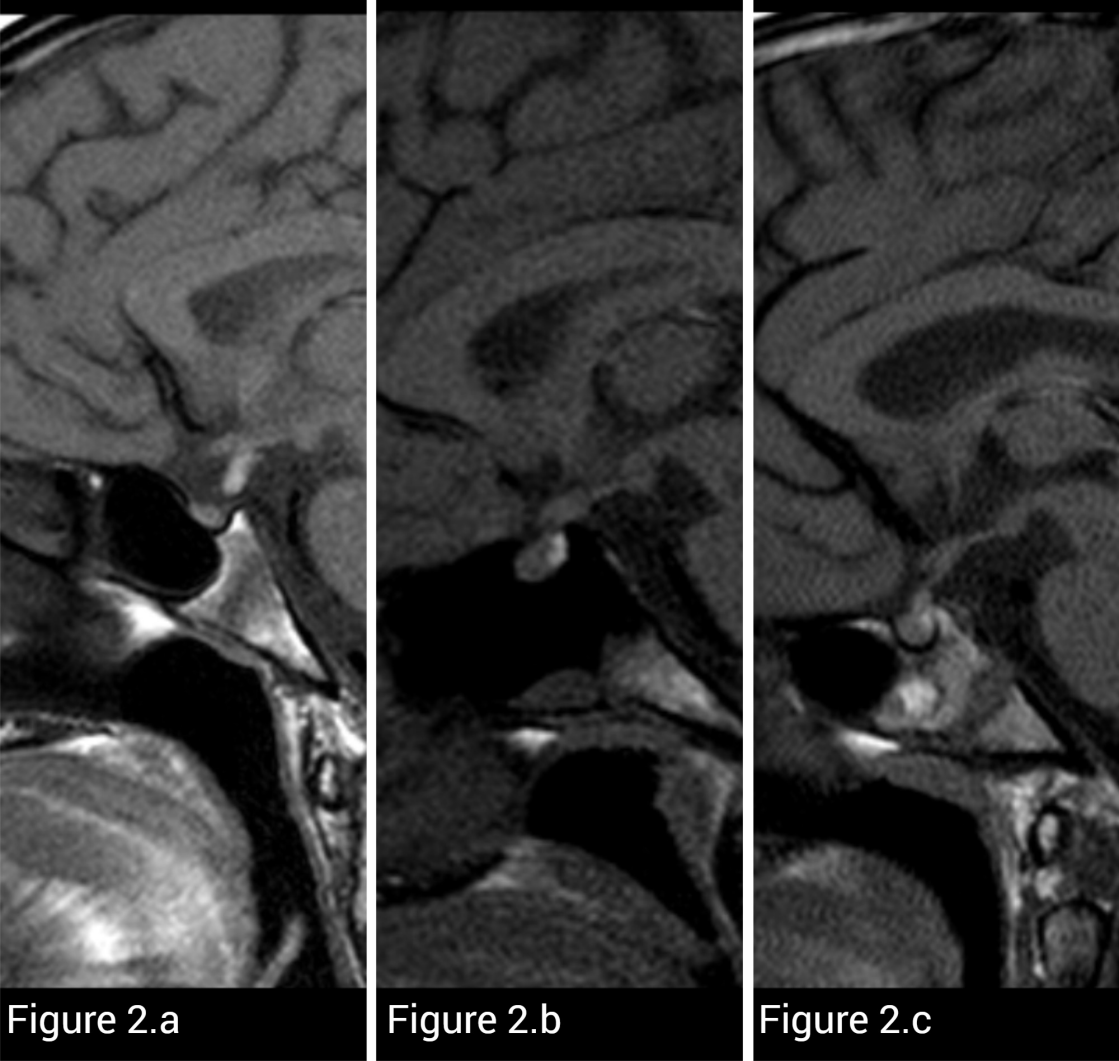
T1-weighted sagittal MR images of three different FA patients. (A) Small pituitary size and high signal corresponding to neurohypophysis located ectopic in pituitary stalk are noted in this patient. (B) MRI shows diffuse thickening of the infundibulum in another patient. (C) This patient presented with nodular thickening of the lower *infundibulum*. It was considered more likely than only thickening of the *pars tuberalis*.
